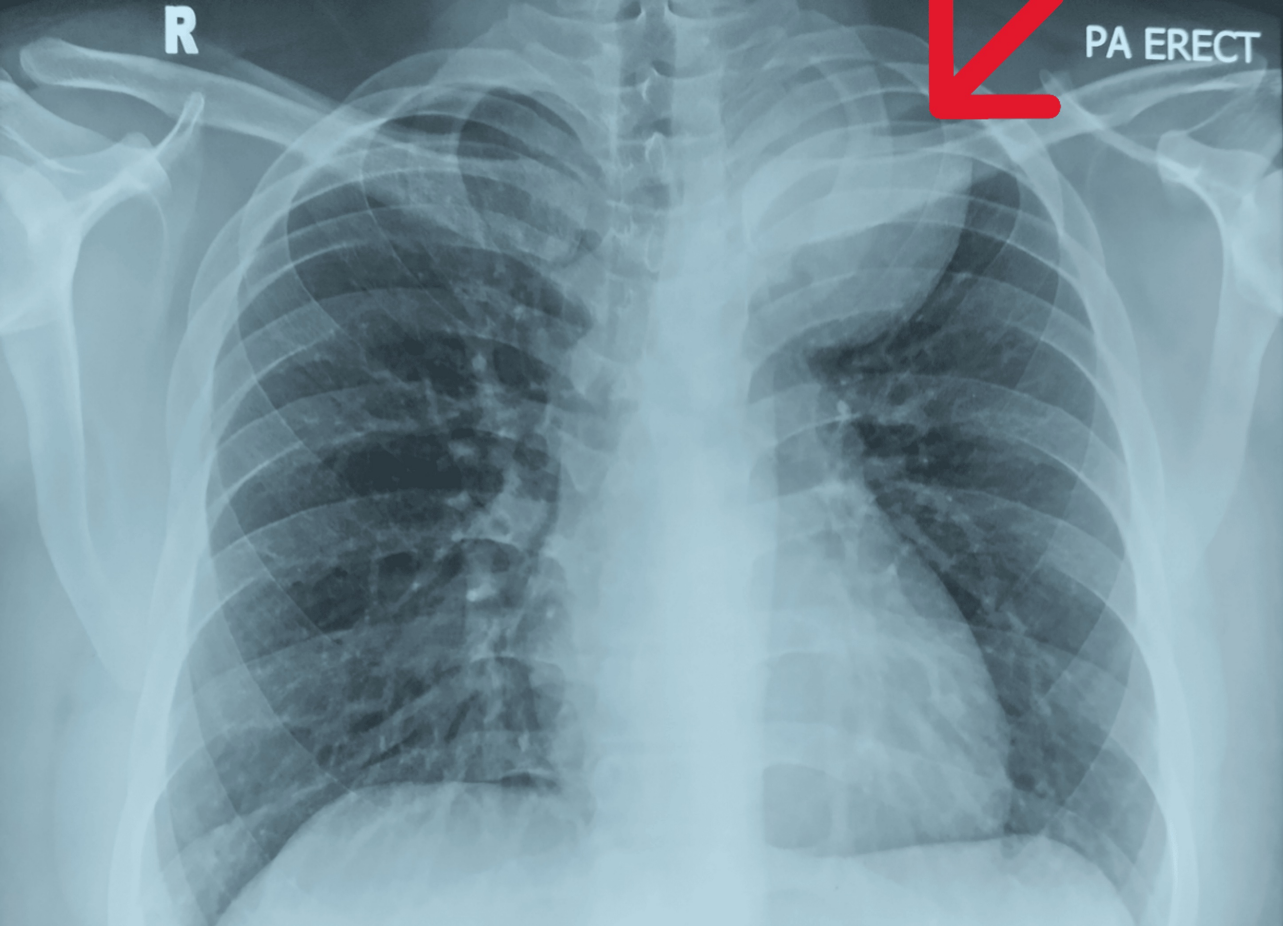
CXR post shoulder reduction. The arrow shows the rounded well-defined mass at the apical left hemithorax. CXR: chest radiograph
